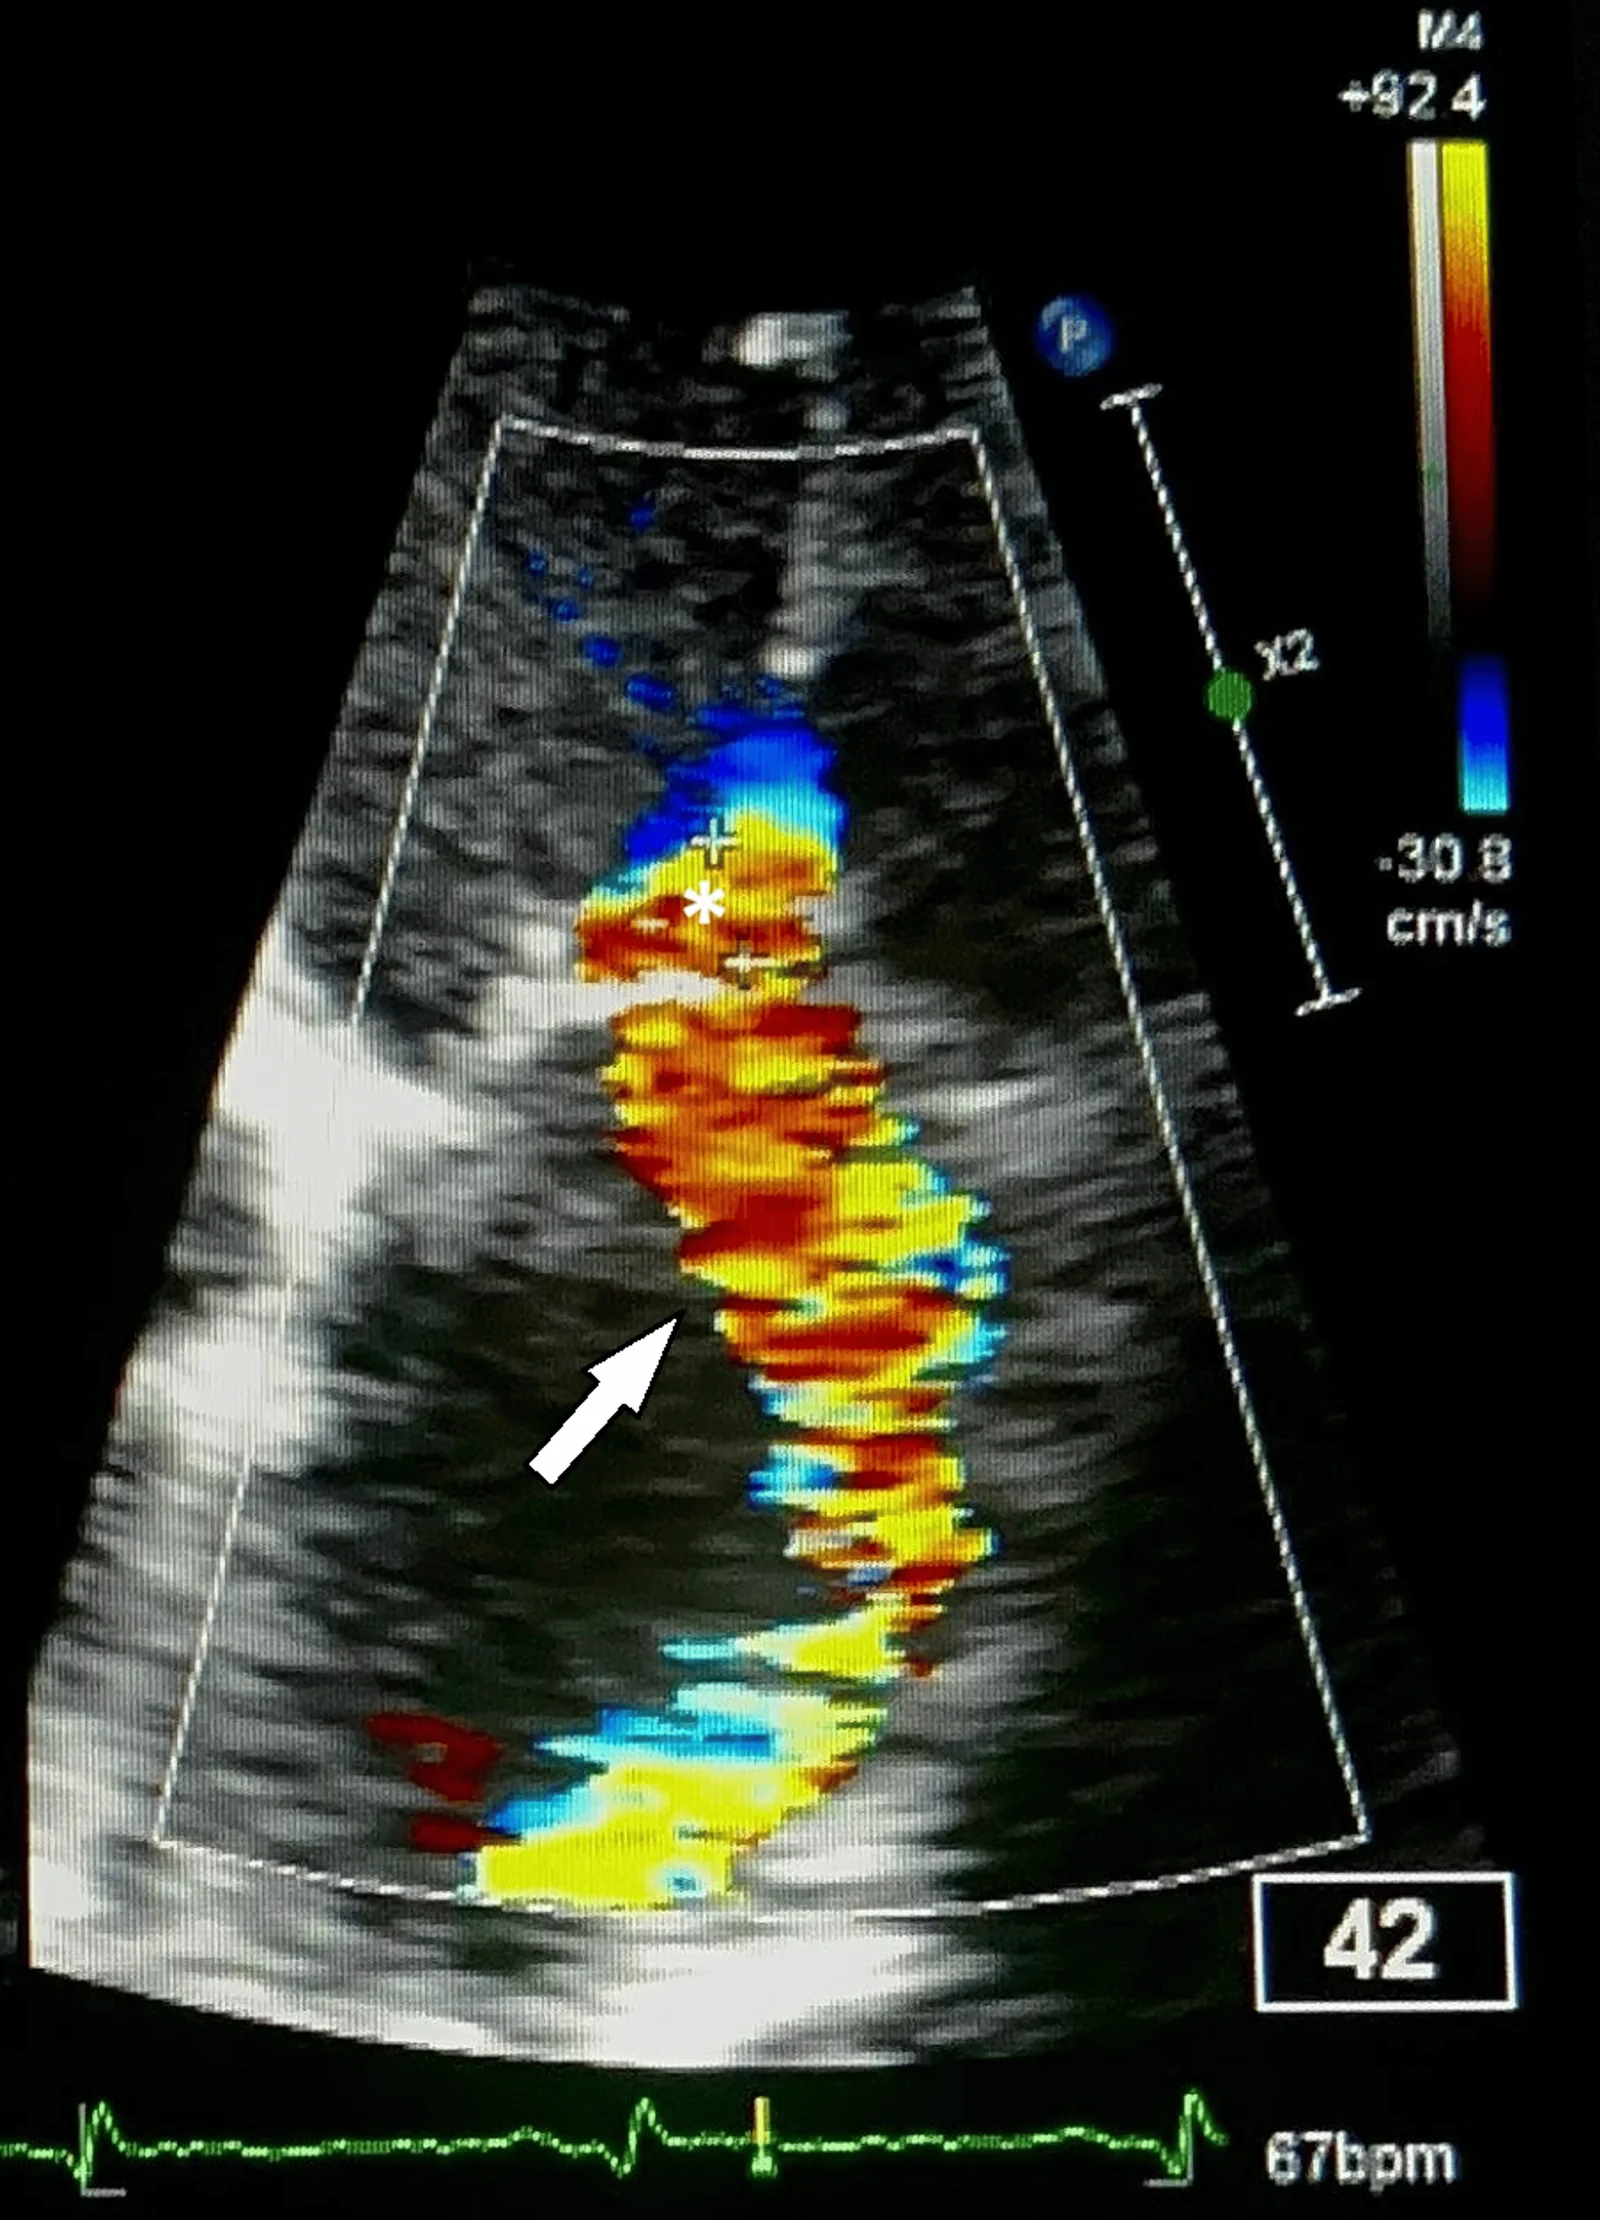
Apical four-chamber color view with focus on trans-mitral flow showing eccentric mitral regurgitation with a posteriorly directed jet (white arrow). MR flow convergence zone (white asterisk) coherent with a Grade III MR. PISA method estimating EROA =0.31 cm², Reg Vol= 45 mL. MR: mitral regurgitation, EROA: effective regurgitant orifice, Reg Vol: regurgitant volume.
